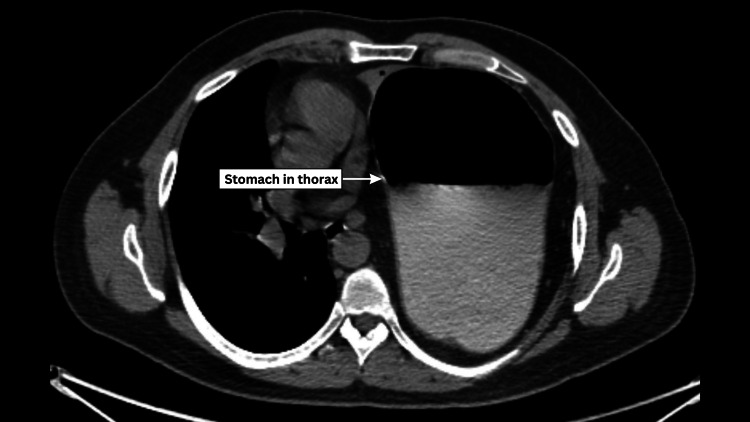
Preoperative axial view of the CT scan showing the whole of the stomach in the thorax
